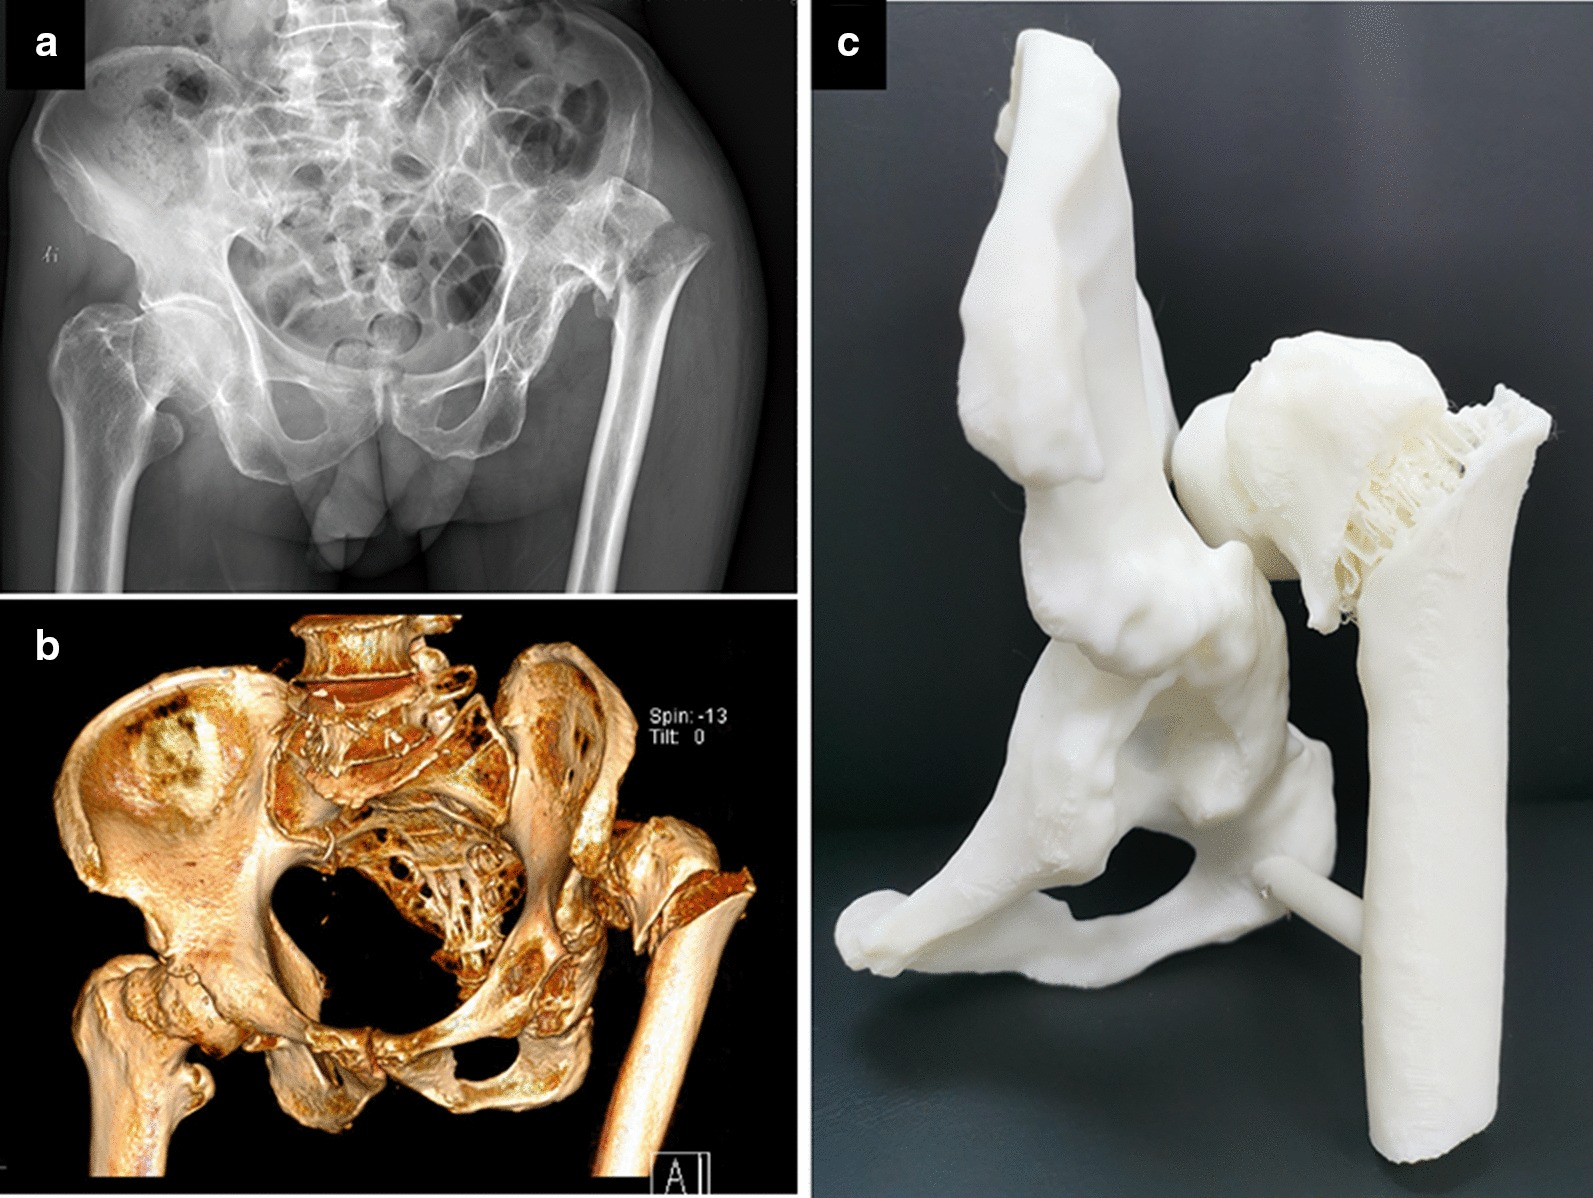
a Preoperative anteroposterior radiographs of the pelvis and hip. b Preoperative 3D computed tomography reconstruction model of the pelvis and hip. c Preoperative 3D-printed model of the pelvis and hip using the computed tomography data
